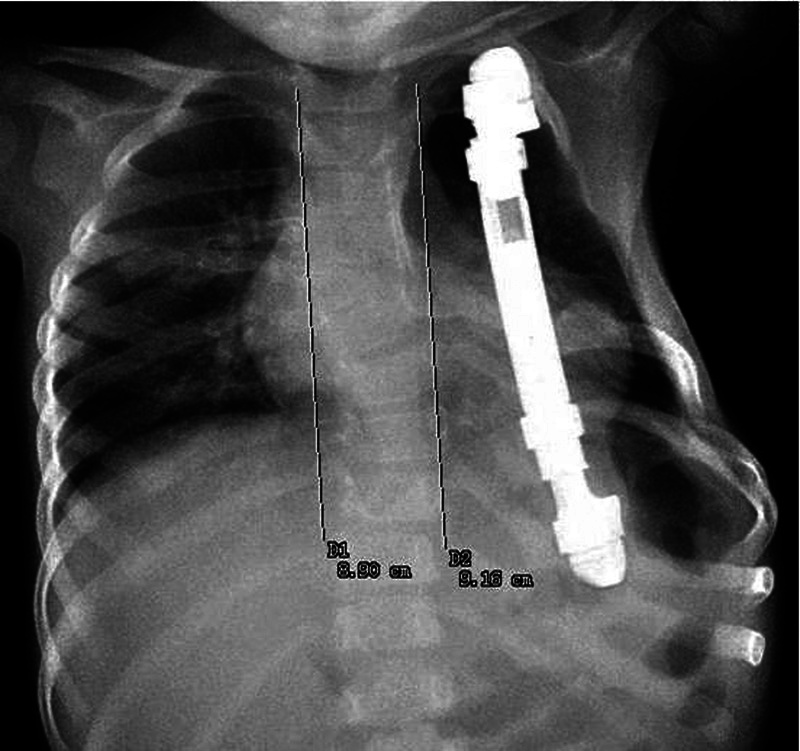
X-ray of the chest 10 months after the operation (anteroposterior view).
